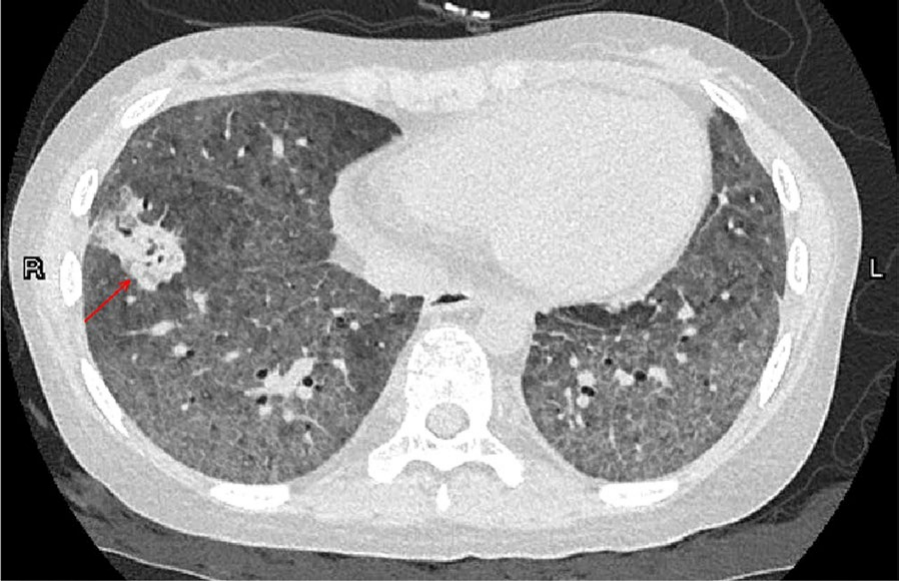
The chest computed tomography (CT) imaging revealed diffuse ground glass changes in the bilateral lungs, partial consolidation(red arrow) and mild bronchiectasis of the anterior basal ganglia in the right lower lobe, and bullae formation in apical segment of upper lobe of left lung
